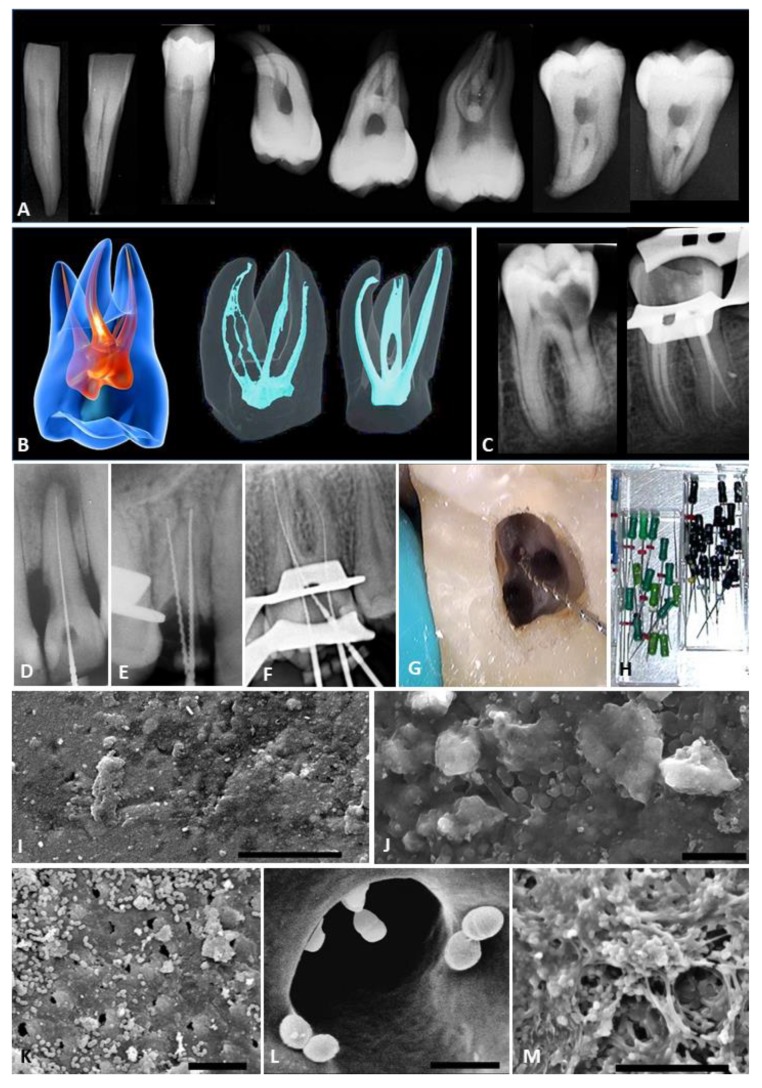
The challenges of traditional endodontic treatment. Panel A shows radiographs (from left to right) of two mandibular incisors, a mandibular premolar, three maxillary molars and two mandibular molars. These are typical examples of internal anatomy of teeth, and show multiple root canals, and complex root curvatures. These root canal systems are difficult to instrument effectively.
